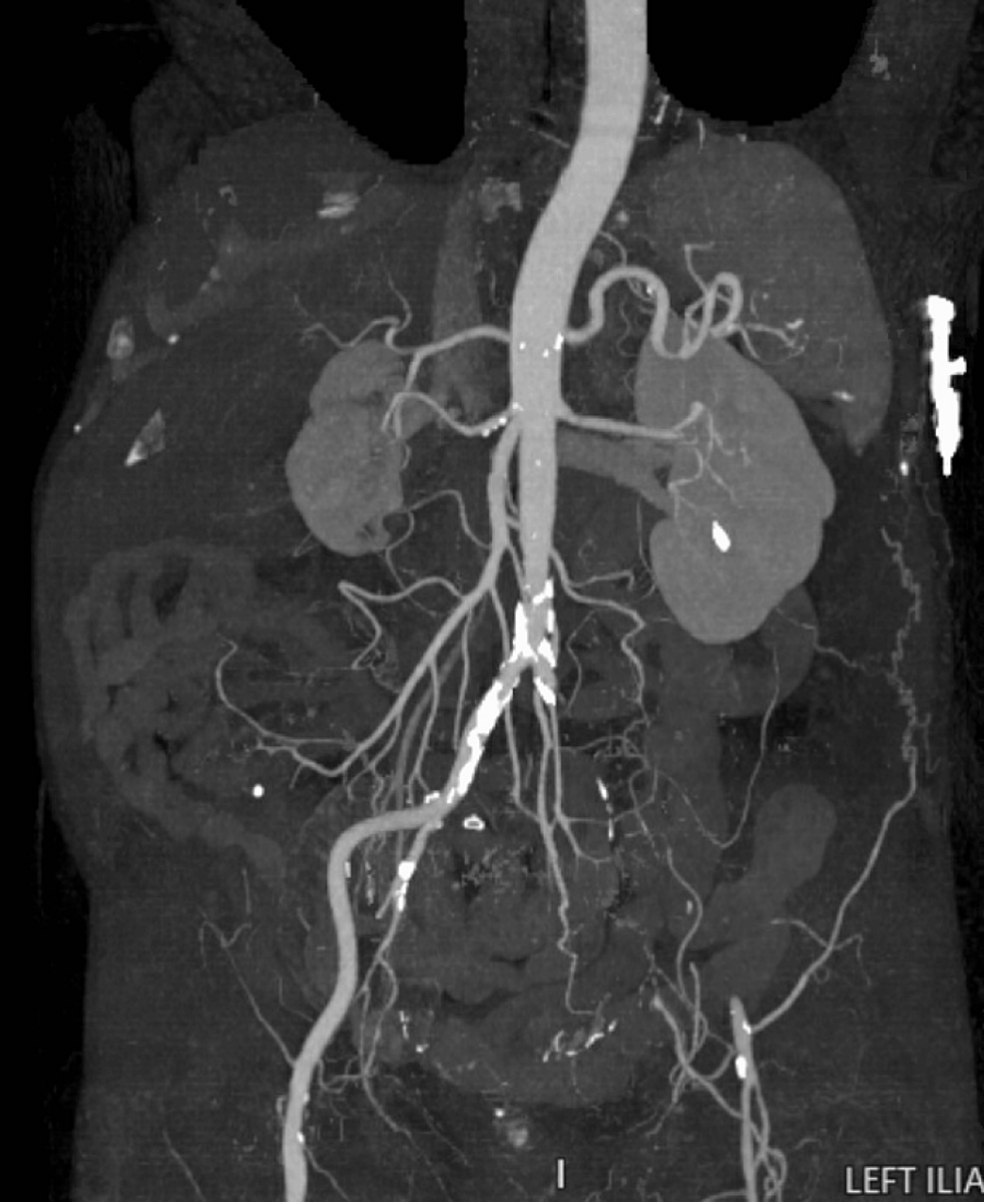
CT angiography imaging demonstrating significant left-sided vascular occlusion
